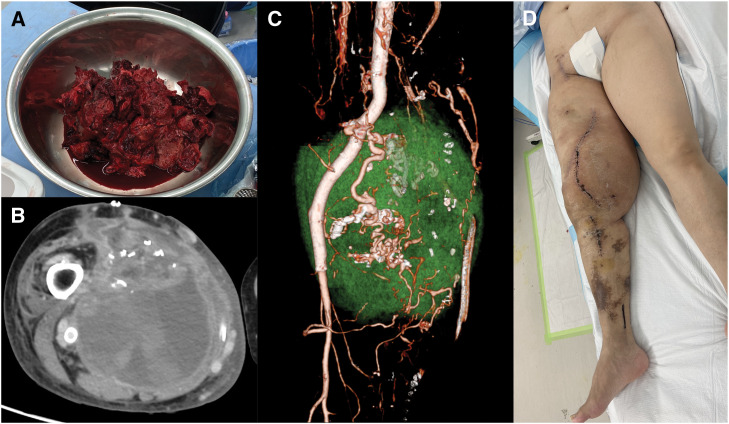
Removed hematoma and the first postoperative findings. The large hematoma was incised, and its contents were removed (A). Postoperative CTA revealed residual hematoma in the distal thigh, raising concerns about potential re-expansion in the future (B). Given the arterial seal achieved with the stent grafts, the remaining arteries were presumed to be osseous origin (C). While the swelling in the thigh improved compared to preoperative levels, the improvement in QOL regarding aesthetic outcomes was limited (D).
